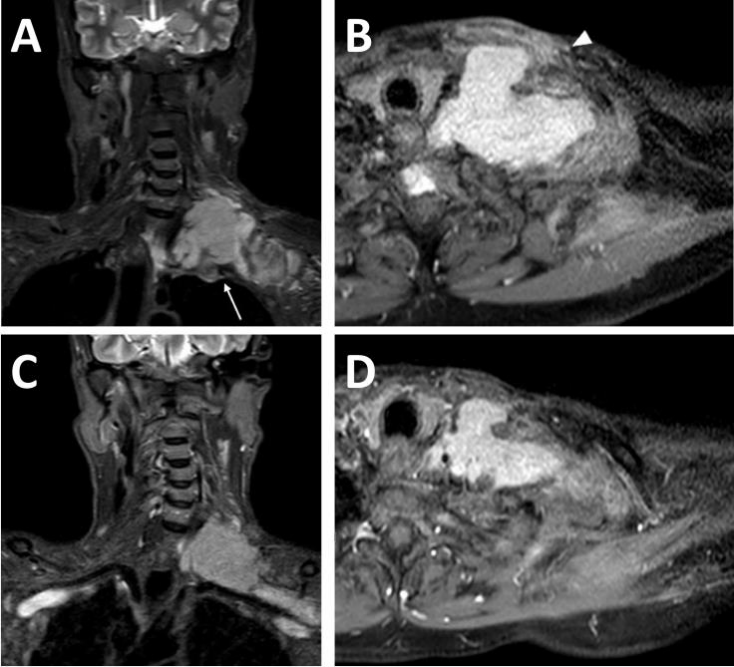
Coronal STIR and Axial Post Contrast MR Images of Neck and Supraclavicular Region Revealing Supraclavicular Swelling in a 55-year-old Patient. A and B: Infiltrative left brachial plexus lesion indenting on lung apex (arrow, A), encasing cervical vessels, with soft tissue edema and enhancement extending up to overlying skin (arrowhead, B) shown on pre-chemotherapy coronal short tau inversion recovery (STIR) (A) and axial post contrast T1-weighted imaging (B). C and D: Corresponding post-chemotherapy images show significant reduction in size of the lesion.
